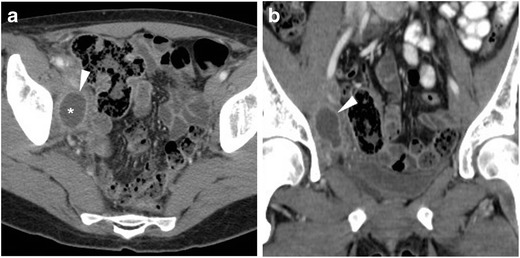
Delayed superinfection of a lymphocele 9 months after radical AH in a 41-year-old female, suffering from recurrent fever after chemotherapy. Contrast-enhanced CT (A, B) showed a fluid-like collection with thick, enhancing wall (arrowheads) in the right lateral pelvis, in the site of a small lymphocele described in a previous ultrasound (not shown). Signs of iliac-femoral thrombosis were absent. Fever, laboratory and imaging abnormalities ultimately regressed after intensive antibiotics (Partially reproduced with permission from ref. no [59])
